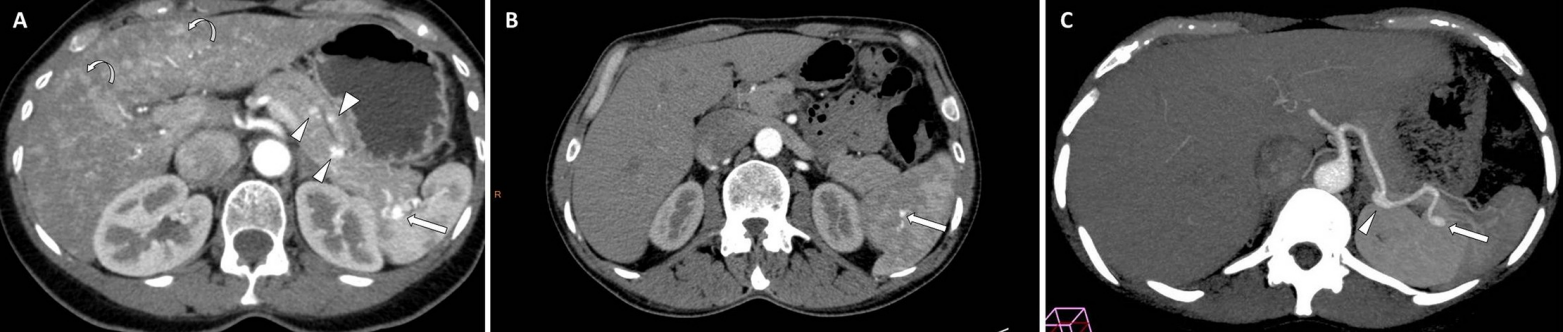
A. SAA in a 52 year-old man with HHT and parenchymal pancreatic involvement. Enhanced computed tomography in axial view show hilar splenic artery aneurysm (arrow) associated with multiple pancreatic telangiectasia (arrowheads). Note the hepatic involvement characterized by heterogeneous enhancement due to multiple hepatic telangiectasia (curved arrows). B. SAA in a 54 year-old man with HHT and a splenic intraparenchymal aneurysm. Enhanced computed tomography in axial view show intraparenchymal splenic artery aneurysm (arrow). C. SAA in a 44 year old woman with multiple locations of SAA (truncular and hilar). Enhanced computed tomography in axial view and maximal intensity projection (MIP) reformation showing an hilar (arrow) and a truncular splenic artery aneurysm (arrowhead).
